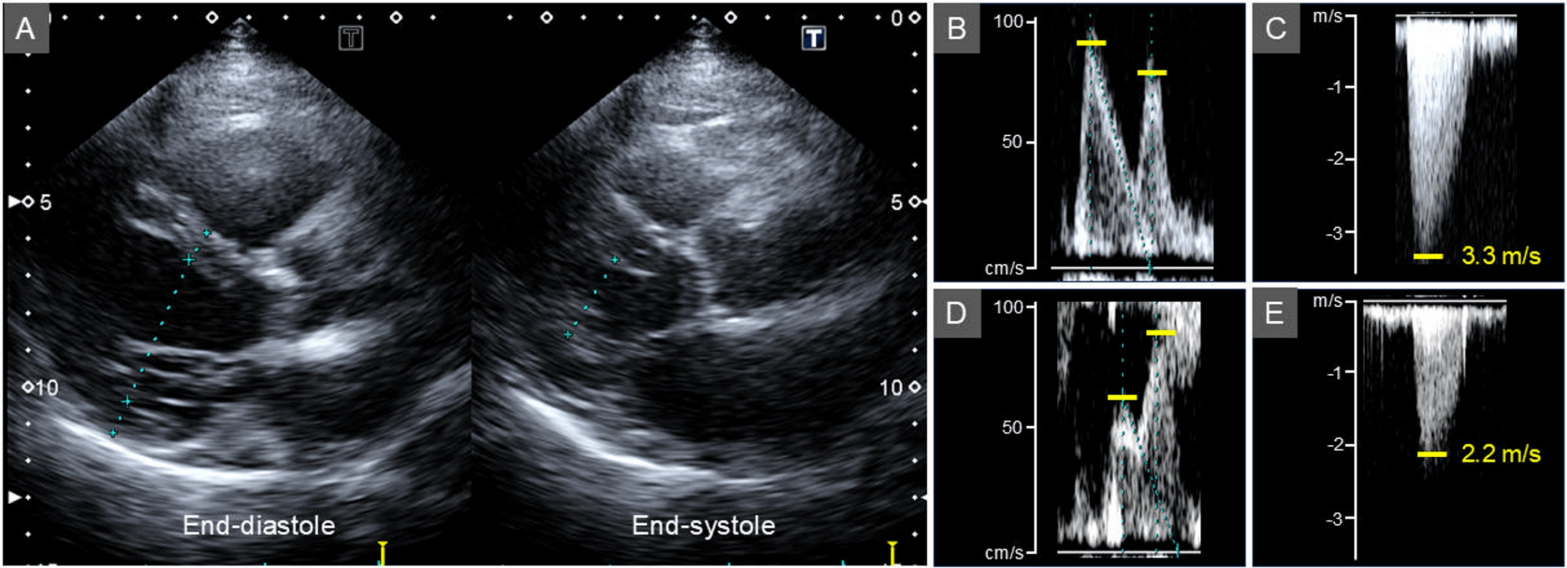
Transthoracic echocardiographic images in case 2. Transthoracic echocardiography revealed a high ejection fraction (EF) of 74% without left ventricular hypertrophy (A), a calcified aortic valve, an early to late diastolic filling velocity ratio (E/A) of 1.2 (B), and a peak aortic jet velocity of 3.3 m/s (C). After thiamine supplementation, EF decreased to 68%, with a change in the E/A to 0.7 (D), and the peak aortic jet velocity declined to 2.2 m/s (E).
